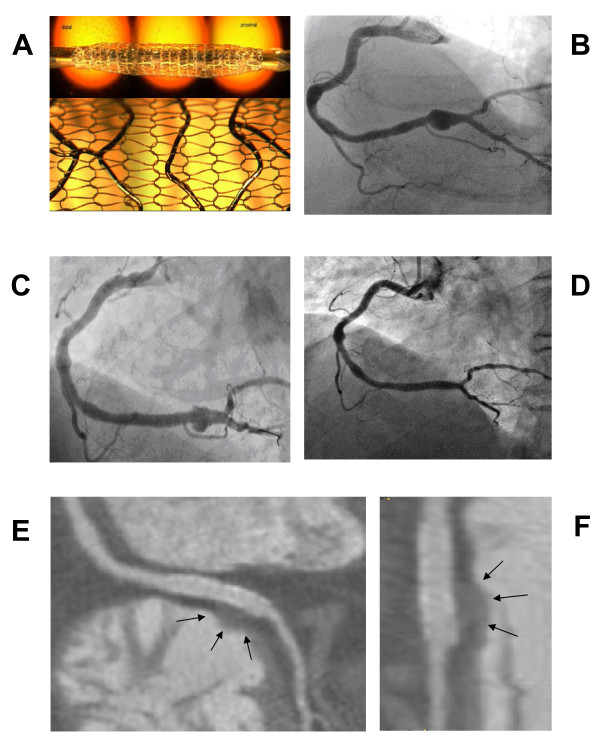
Coronary angiography and CT scan before and after MGuard stent implantaion. (A) The unexpanded (top) and the expanded stent covered with a sleeve mesh (bottom). Reproduced with permission from InspireMD. (B) Angiography of the right coronary artery with the presence of a large saccular aneurysm involving the distal part of the artery to the crux cordis. (C) Partial opacification of the aneurysmal sac through the holes of the mesh just after stent implantation. (D) Coronary angiography at one month follow-up showing the exclusion of the aneurysm. (E) Coronary CT scan at one month: multiplanar reformation of the right coronary artery near the crux cordis; on the right ventricle side of the distal part of the stent, is clearly demonstrated the water density remnant of the treated aneurysm (arrows): low density fat is surrounding the proximal stent. (F) Coronary CT scan at one month: The magnified view of the stent allows for a better identification of the treated aneurysm (arrows).
